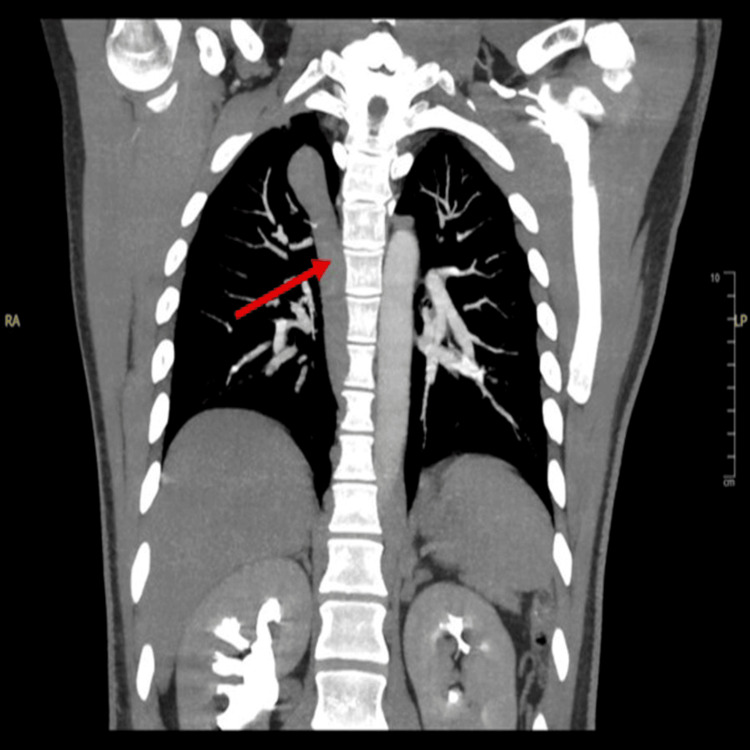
Contrast-enhanced CT of the abdomen demonstrating prominent collateral venous circulation through the azygos/hemiazygos system CT: computed tomography
